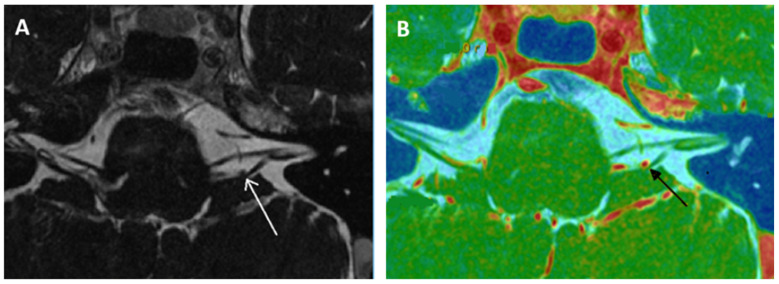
Encephalic MRI with IAC-centered slices. (A): T2 high resolution. Mass effect by the left posterior–inferior cerebellar artery (white arrow) on the cisternal path of the left vestibular nerve (in the root entry zone) with slight deviation. (B): High resolution MRI in axial plane fused image between 3DT1-weighted contrast enhanced sequence and 3DT2 DRIVE sequence. This type of sequence enables better analysis of the vascular–nervous contact (black arrow).
